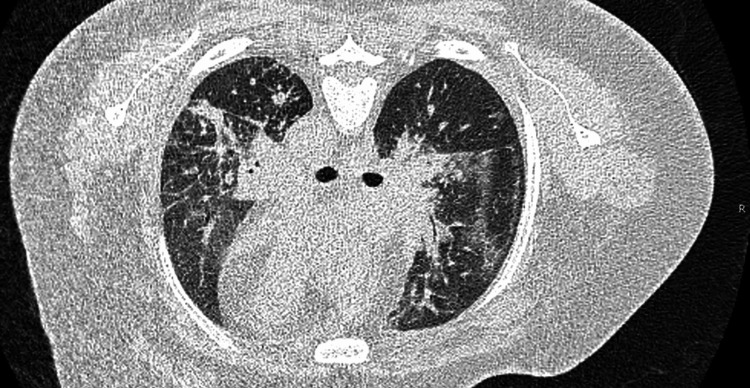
High-resolution CT of the chest without contrast High-resolution CT of the chest on day 4 indicating multifocal areas of airspace consolidation in each lung, most consistent with post-obstructive pneumonitis/atelectasis.
